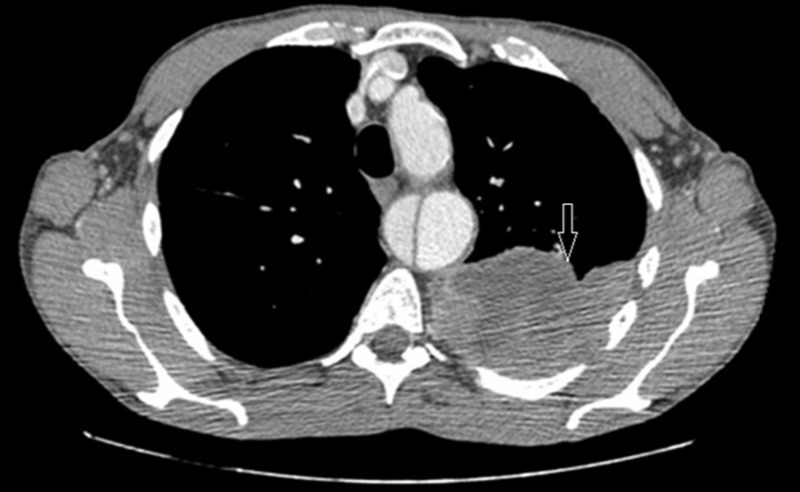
Computed tomography (CT) chest with contrast - axial section The arrow shows a 21.5 x 9 x 10.2 cm mass in the posterior mediastinum.
